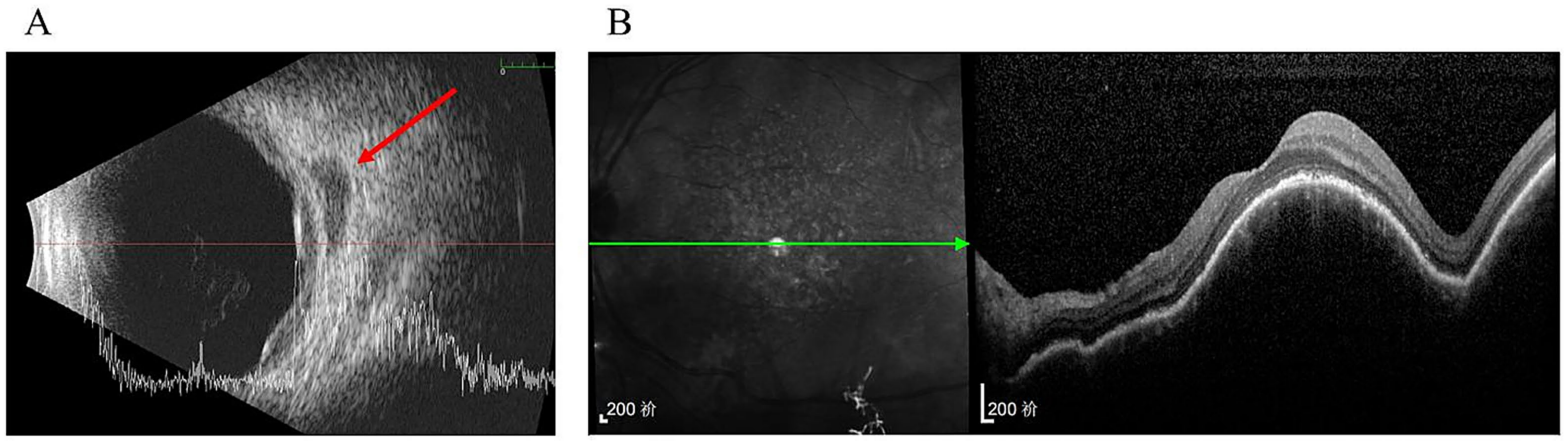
Three months after the initial presentation. (A) Ultrasonography showed the area of diffuse choroidal thickening and retrobulbar hypoechoic area were slightly increased (arrow); (B) No significant change in the performance of OCT compared with the presence of last time.
